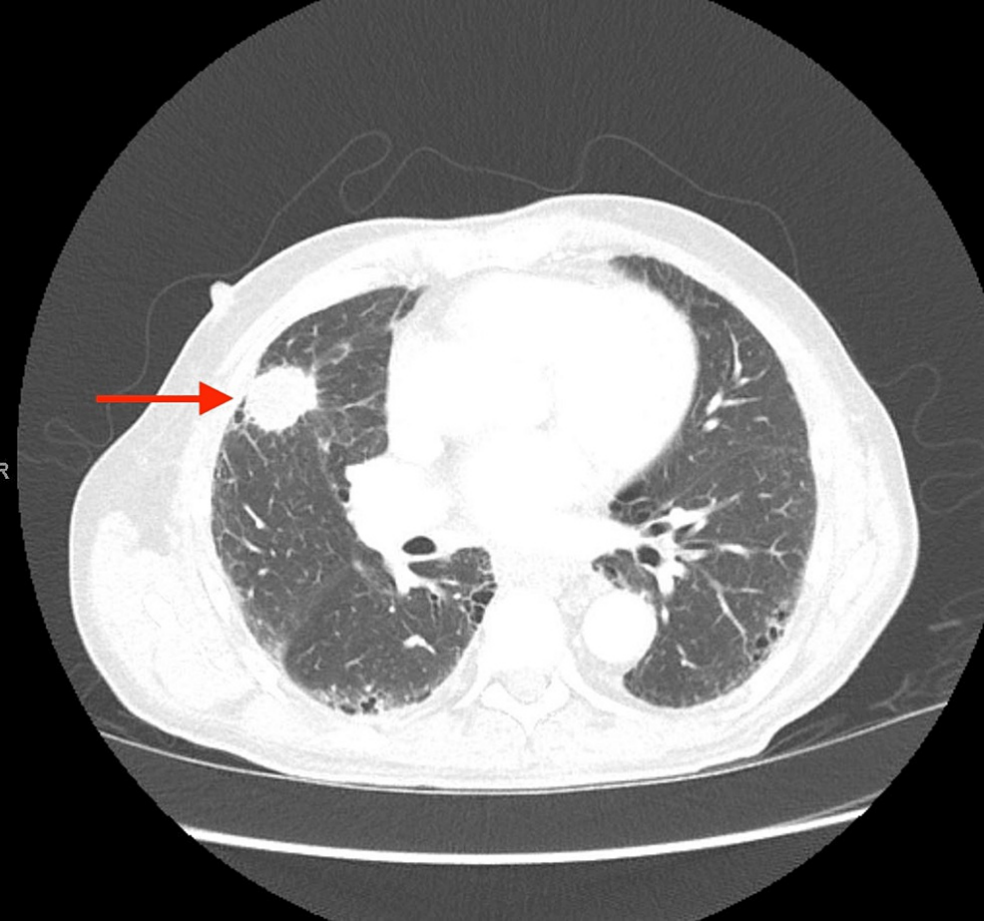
Axial chest CT demonstrating the presence of a right upper lobe spiculated mass (arrow).
